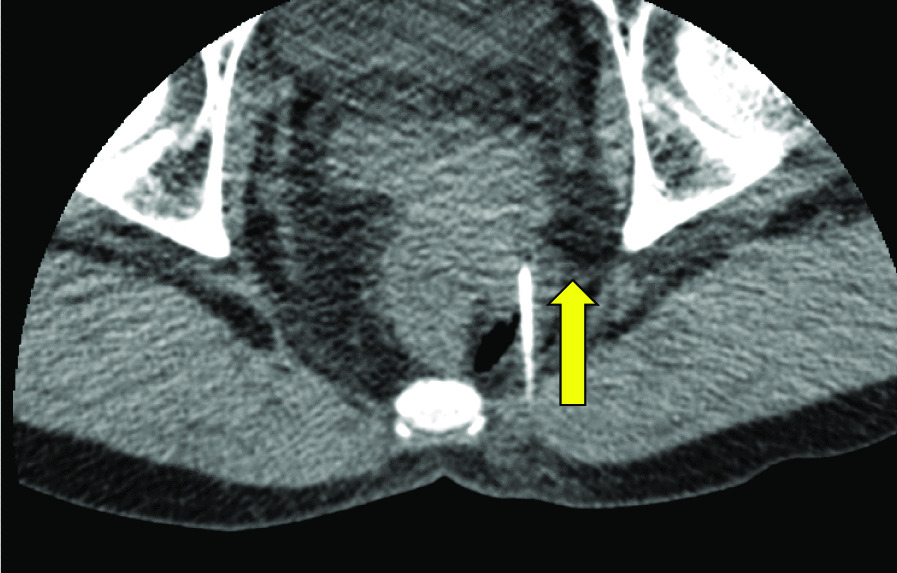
CT-guided percutaneous needle biopsy. The yellow arrow indicates the direction of the biopsy. CT, computed tomography
